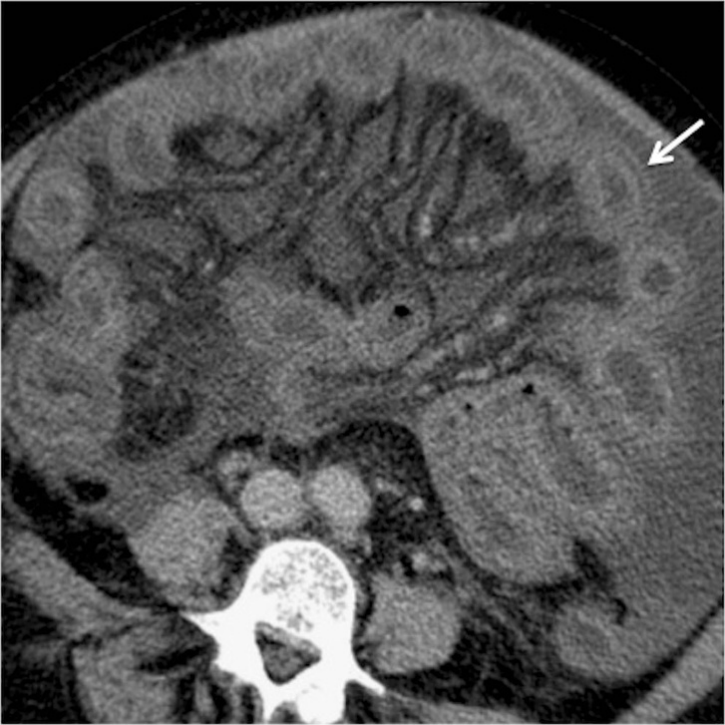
Acute small bowel ischaemia. Axial contrast-enhanced CT scan shows diffuse thickening of the small bowel loops with a target appearance (*arrow*) due to submucosal oedema. Also note the engorgement of the mesenteric root vessels and ascites, common findings in cases of acute bowel ischaemia. *Adapted from the electronic poster “Bowel wall thickening—a complex subject made simple” DOI:*10.5444/esgar2011/EE-063
